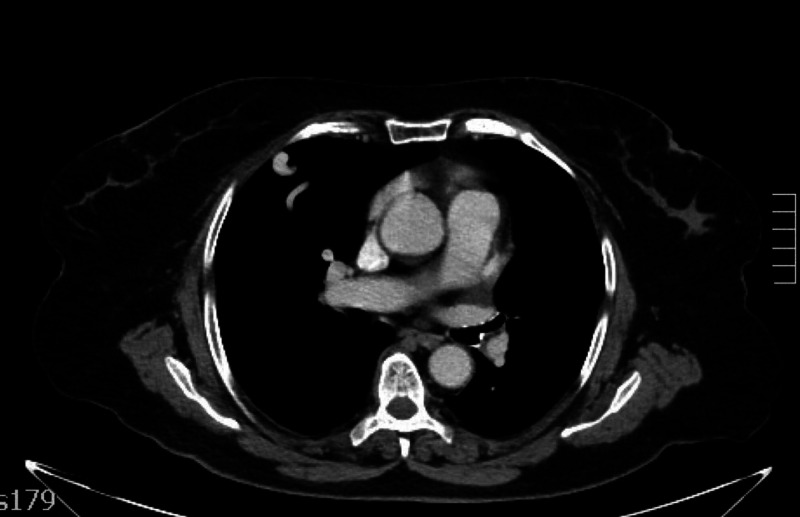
Contrast chest CT axial images shows a PAVM as a plexiform mass of dilated vascular channels. PAVM, pulmonary arteriovenous malformation
